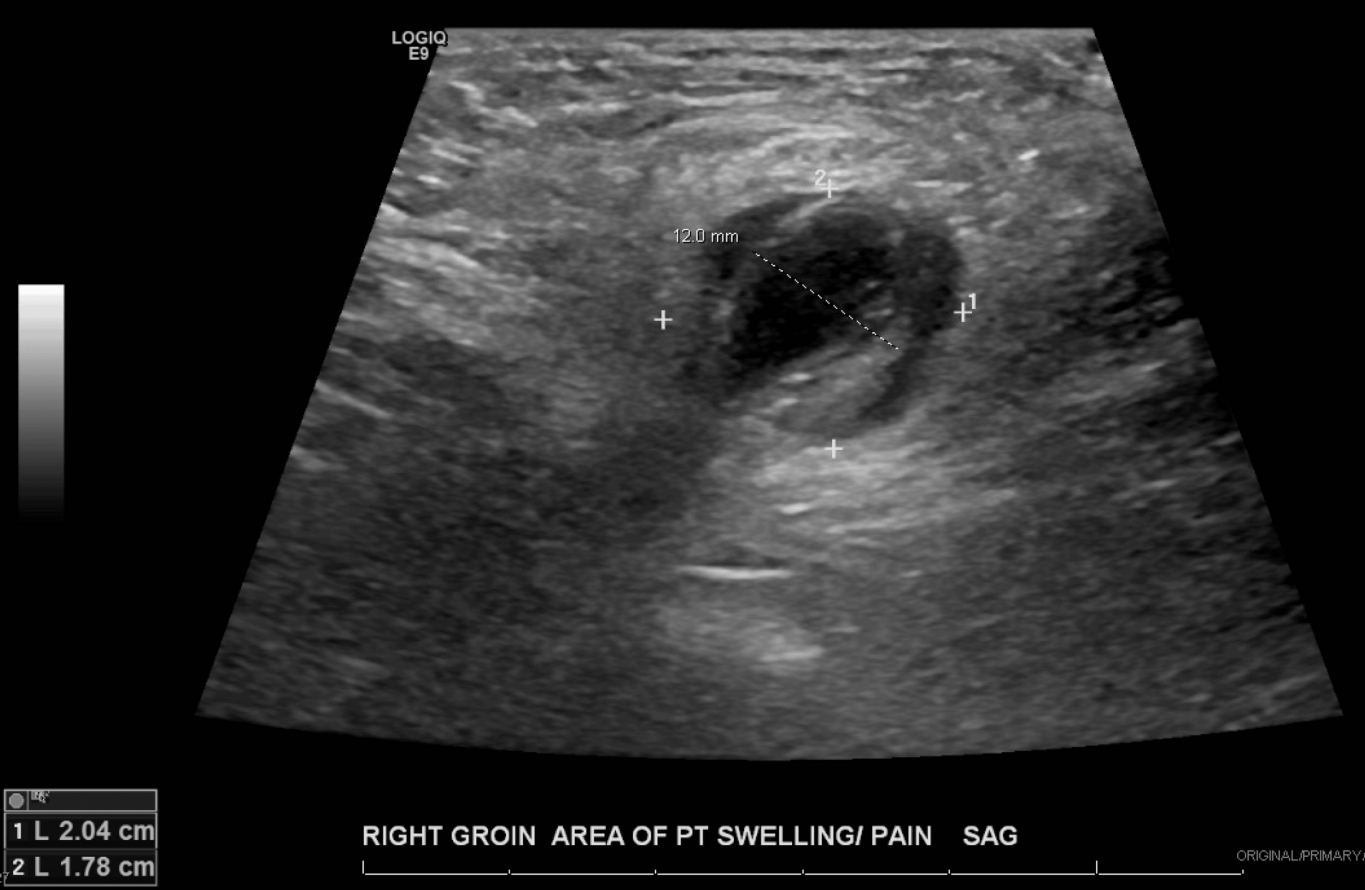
Ultrasound of the right groin Sagittal view of the hernia, which measures 2.04×1.78 cm, containing the appendix, which measures 12 mm.
